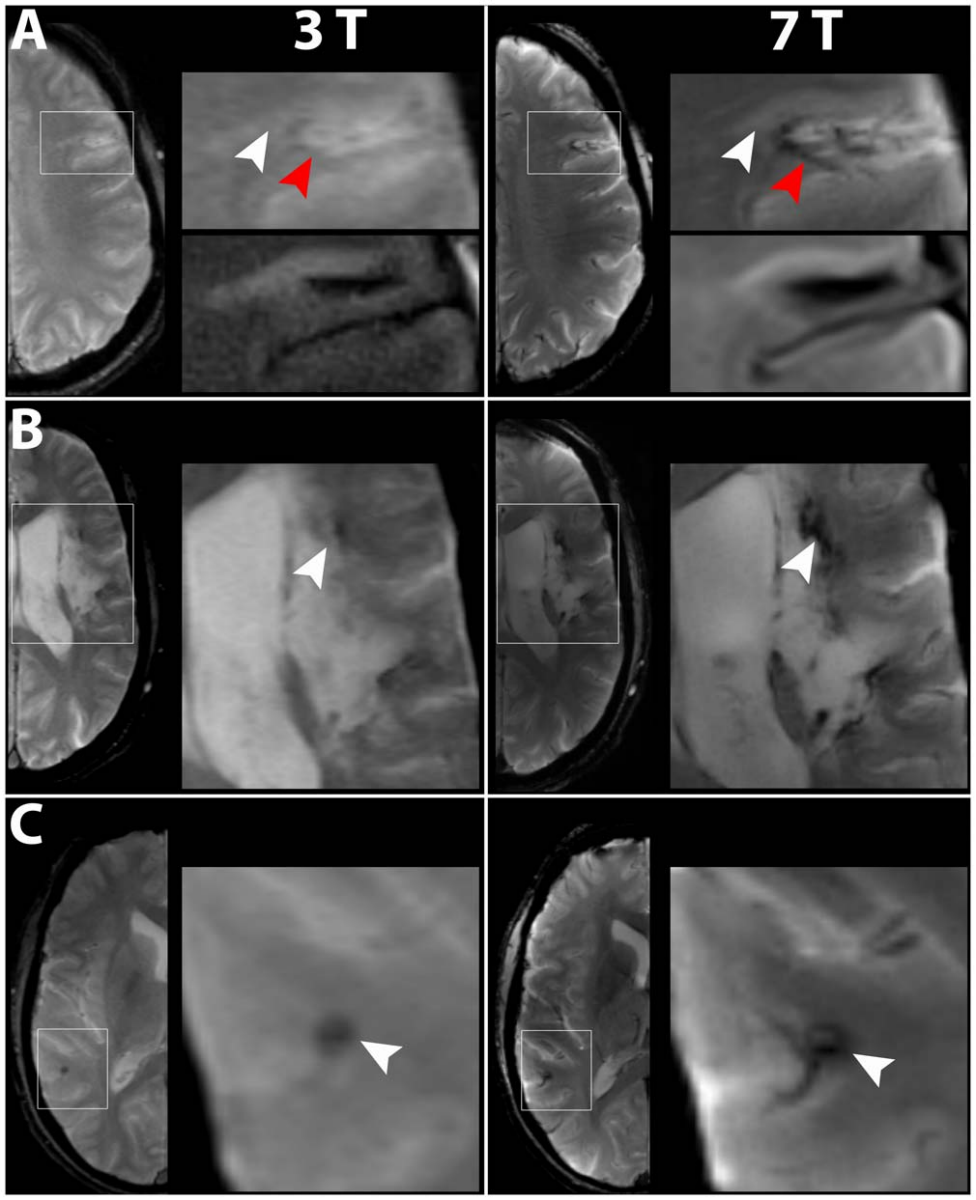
Comparison of T2*-weighted images acquired with HemoFLASH at 3 T and 7 T. In all patients, HemoFLASH provided higher anatomic detail level at 7 T. Moreover, hypointense perilesional hemosiderin deposits were much more pronounced at 7 T. Boxed areas are shown at higher magnification. A) In patient No 3, anatomical detail level and contrast of the lesion (white arrowheads) to healthy tissue were higher at 7 T imaging. T2-FLAIR weighted high magnification images are shown for comparison below. A perilesional hypointense area, indicating hemosiderin deposits, was much more pronounced at 7 T (red arrowheads). B) In patient No. 4, again both anatomical details as well as the imaging of hemosiderin (white arrowheads) were superior at 7 T. C) Incidental finding of a cavernous angioma (white arrowhead) in patient No. 5. The internal structure of the lesion, showing a nodular characteristic with a hypointense rim, and the depiction of feeding vessels were more pronounced at 7 T, facilitating the diagnosis.
